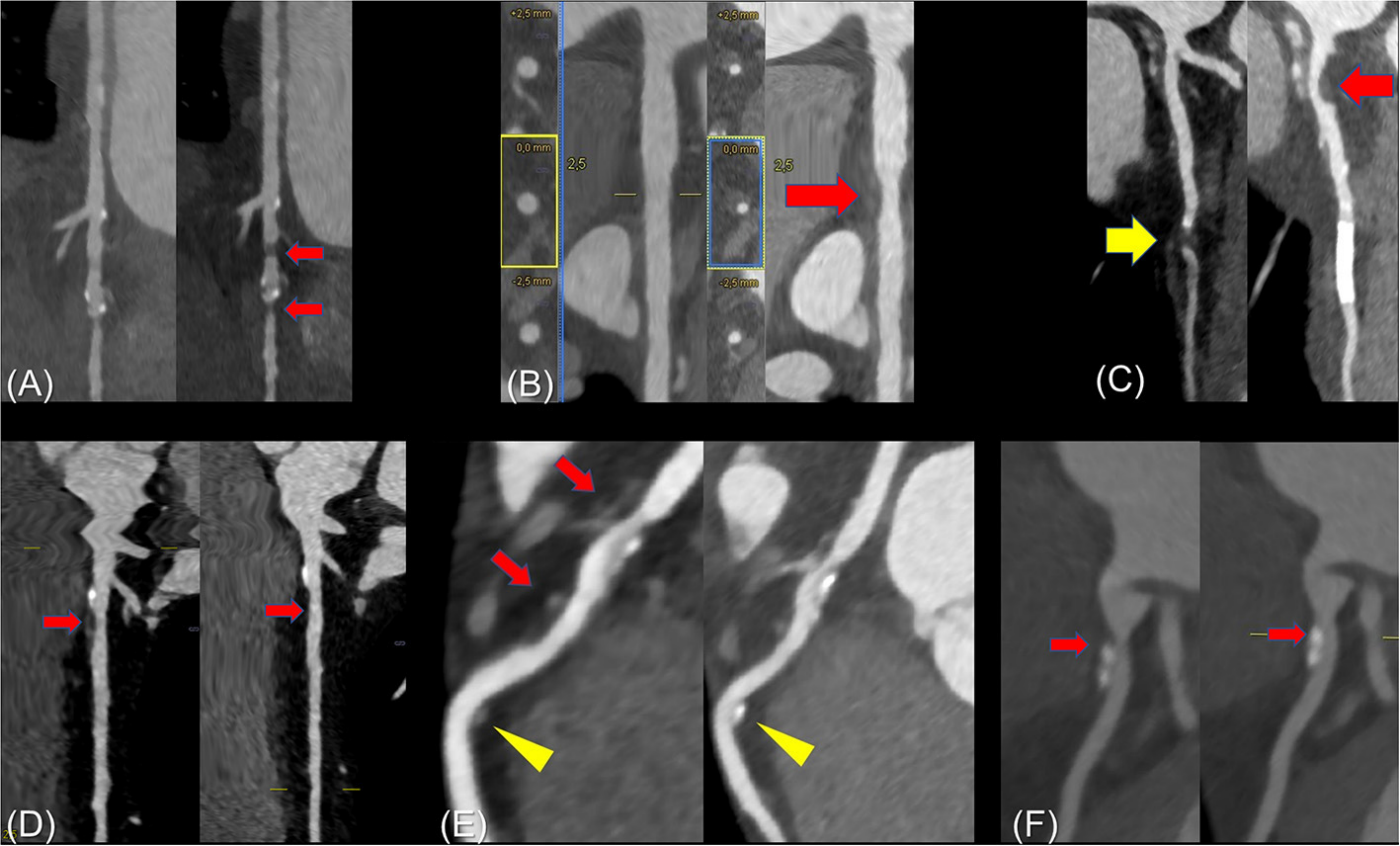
Examples of progressive and regressive plaques. (A) Rapidly progressive CAD with the occurrence of two significant plaques in the distal LCX within a period of 14 months. (B) Initially, only slight irregularities of the proximal LCX were found. Statin therapy was rejected by the patient. As a malignant thymoma was incidentally found at the combined coronary and thoracic CT examination, the patient underwent radiotherapy, which contained the proximal LCX in the radiation field. In the follow-up CTA examination after 18 months, a non-calcified plaque with a lumen narrowing of ≈50% was found in the LCX with a new inflammatory border in the perivascular fat tissue (arrow). (C) The initial coronary CTA showed a short-length closure of the LAD segment 7 (yellow arrow) and slight wall irregularities proximally. The follow-up examination after 44 months demonstrated an open coronary artery stent and a significant progression of atherosclerosis with a new non-calcified plaque in the proximal LAD segment 6 (red arrow). (D) Initially, the proximal LAD showed calcifications with an adjacent non-calcified plaque with negative remodeling resulting in a mild stenosis. After 18 months of intensified statin therapy, almost complete regression of the vulnerable plaque with only slight wall irregularities of the vessel wall was observed. Of note, even the soft hem around the proximal calcification was not detectable any longer. (E) The predominantly non-calcified plaque (red arrows) with positive remodeling and fibrous cap diminished clearly in the follow-up coronary CTA after 36 months of statin therapy. The distal non-calcified plaque (yellow arrows) almost calcified completely. (F) The initial examination showed a dumbbell-like calcification with surrounding soft tissue cover (arrow) of the LAD. After statin therapy for 24 months, an increasing calcification was detectable and the apical non-calcified plaque did not longer appear. The vessel area increased from 2.9 mm^2^ to 3.8 mm^2^.
